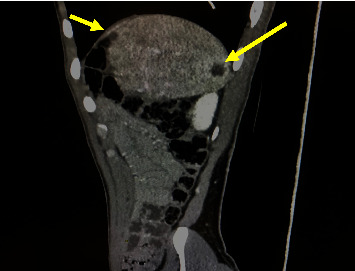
Abdominal spiral CT scan with contrast shows a few near together cystic lesions with faint enhancement after administration of contrast (arrows).
